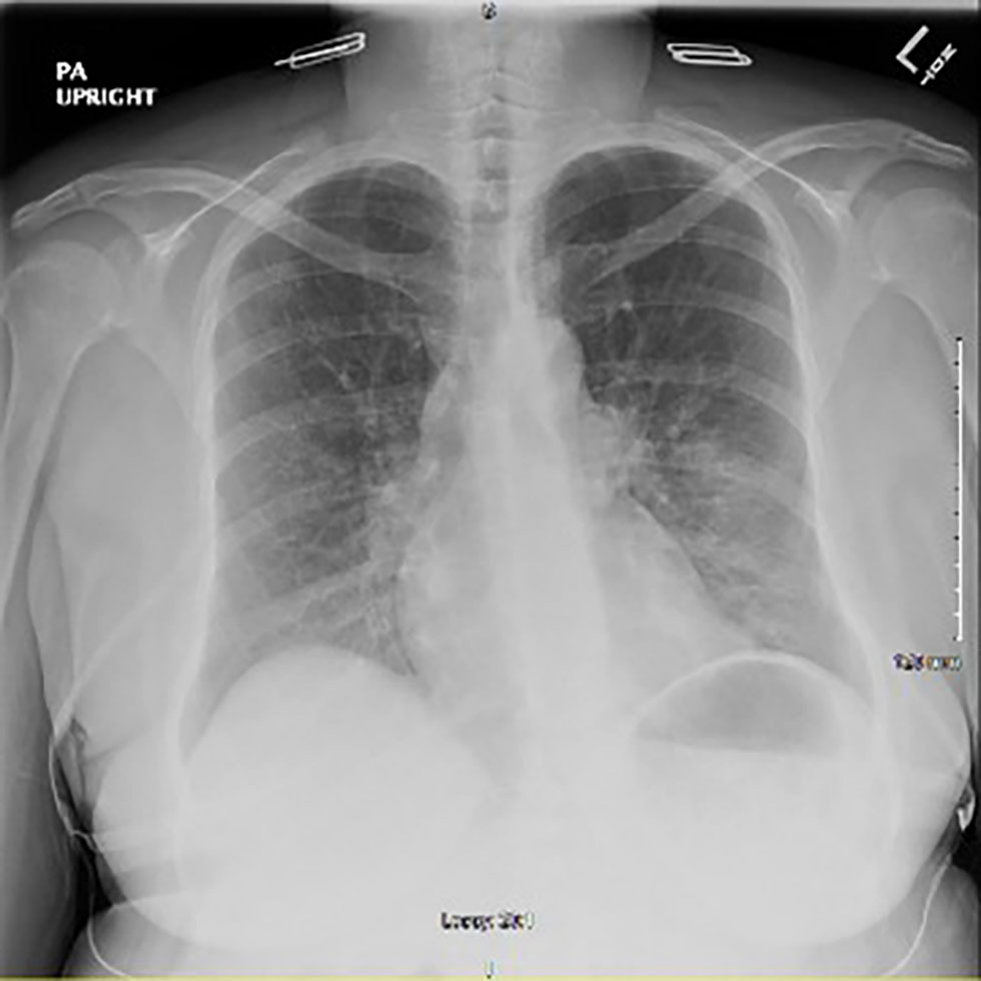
Initial chest x-ray after onset of symptoms showing peribronchial vascular opacities in the left lung base and left perihilar region.
